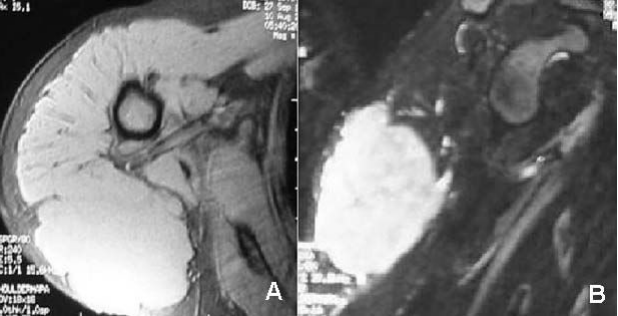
MRI images of the lesion. A) Axial MRI performed with T1 gradient-eco sequence. B) TSE sequence with deletion of the T2 signal from adipose tissue.
